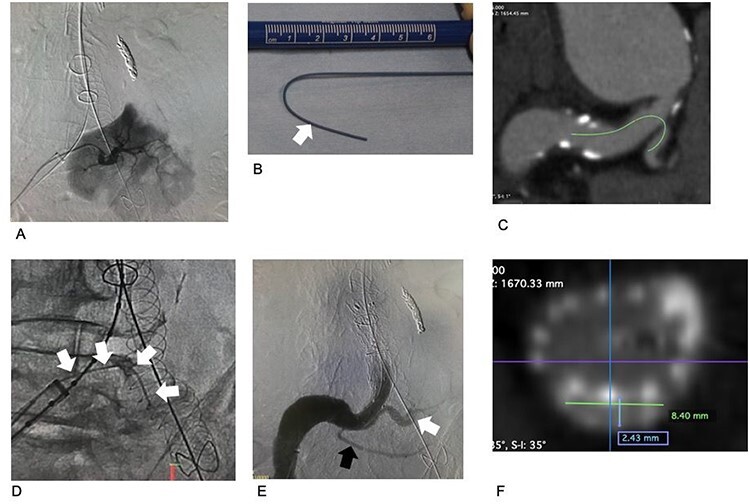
Images associated with chimney graft technique procedures. (A) Selective arteriogram of the third renal artery. The area perfused by the artery is large. (B) Before insertion, a stiff wire was shaped according to the preoperative CT image analysis. The tip of the stiff portion is indicated by a white arrow. The portion distal to the white arrow is more pliable, preventing damage to the third renal artery and the kidney. (C) Preoperative CT. The estimated access route for the chimney graft and the stiff wire is indicated by a green line. (D) The stiff wire is placed into the third renal artery. (E) Arteriogram after completing all procedures. The third renal artery is perfused via the covered stent (white). The fourth renal artery is preserved (black). No gutter-related type II endoleak occurred. (F) Postoperative CT. The cross-section of the chimney graft is 2.4 × 8.4 mm.
